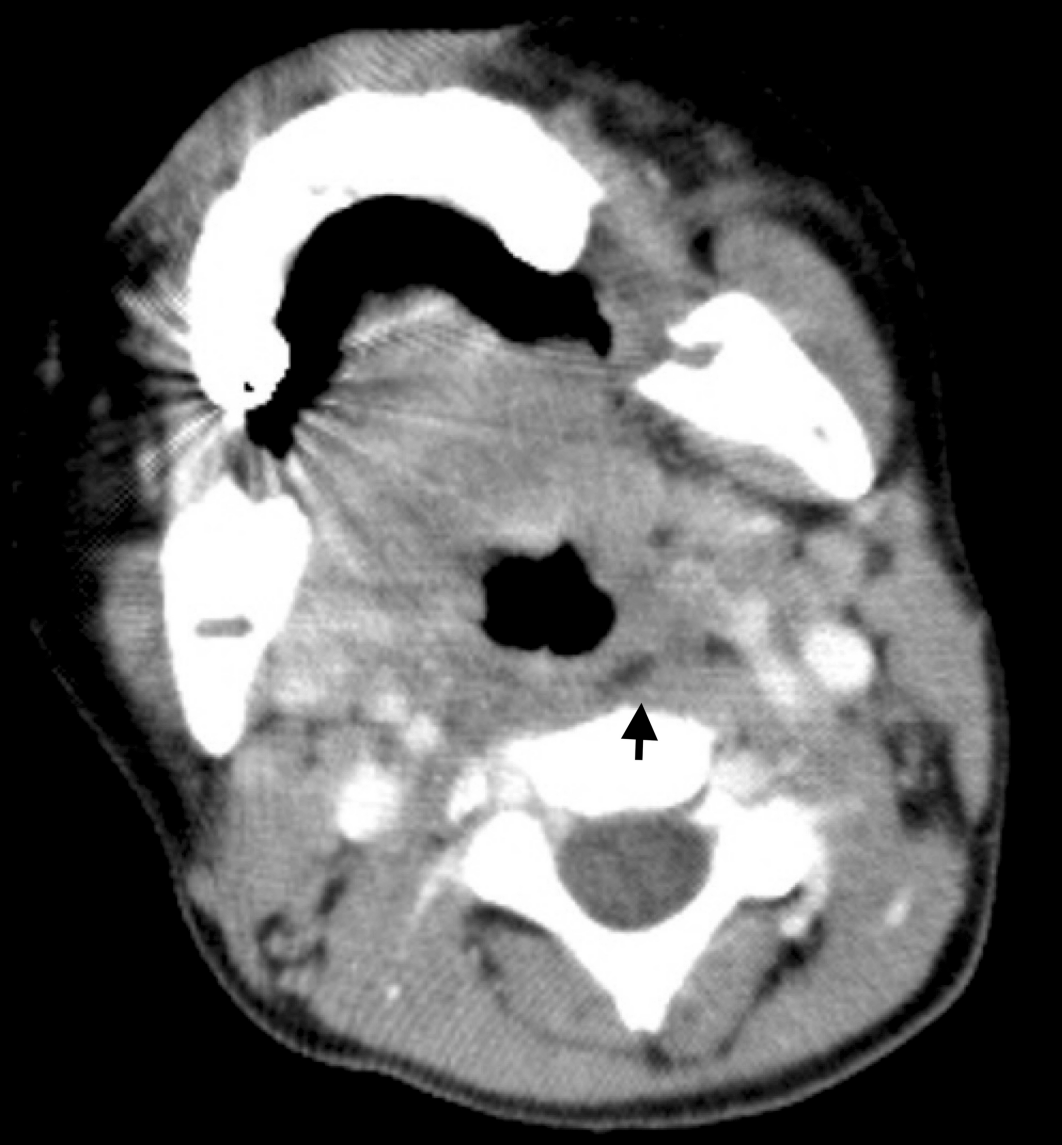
Enhanced computed tomography of the patient's neck revealed retropharyngeal edema. The black arrow indicates edema in the posterior wall of the pharynx.
